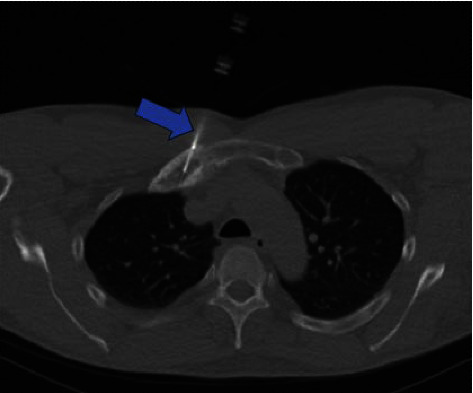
Axial CT-fluoroscopic image of the upper thorax during percutaneous biopsy of the right first rib chondral abnormality using a 13-gauge bone biopsy needle (blue arrow).
